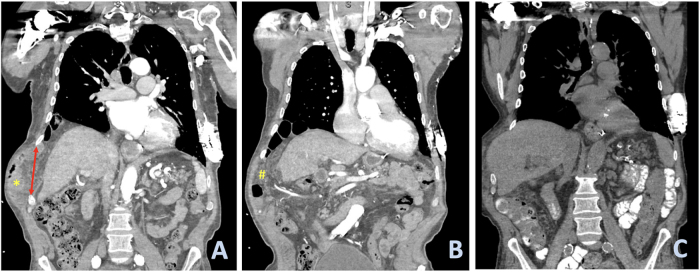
Pre- and postreduction CT scans. Herniation of bowel (* in image A) and omentum (# in B) into the right hemithorax, with associated bulging of the right thoracic wall and widening of the 10th intercostal space (double arrow in A). After spontaneous reduction of bowel and omentum, herniation of the liver into the right hemithorax through the widened 10th intercostal space is visible (C).
